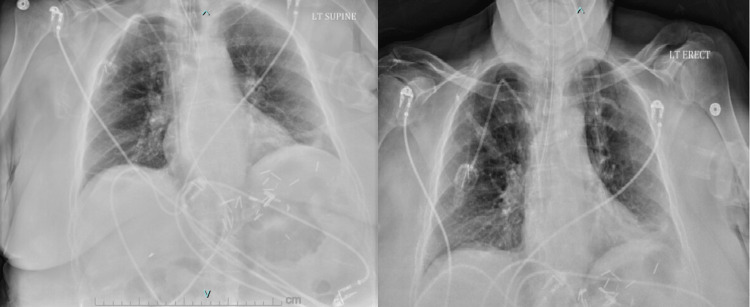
A normal chest X-ray shows no cardiopulmonary abnormalities.
